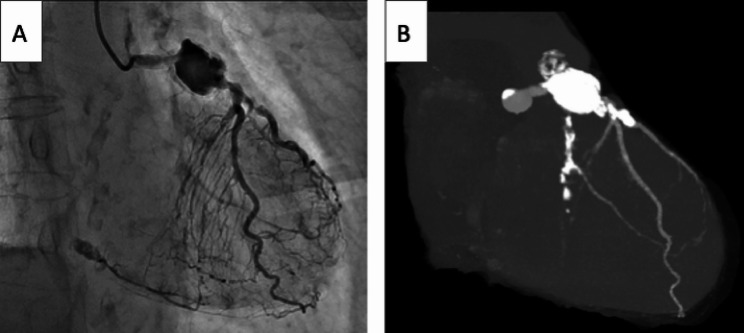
Preoperative imaging. (A) Coronary angiography showing a giant aneurysm of the proximal left anterior descending artery. (B) Three-dimensional computed tomography reconstruction demonstrating the 20-mm aneurysm and coronary artery anatomy
